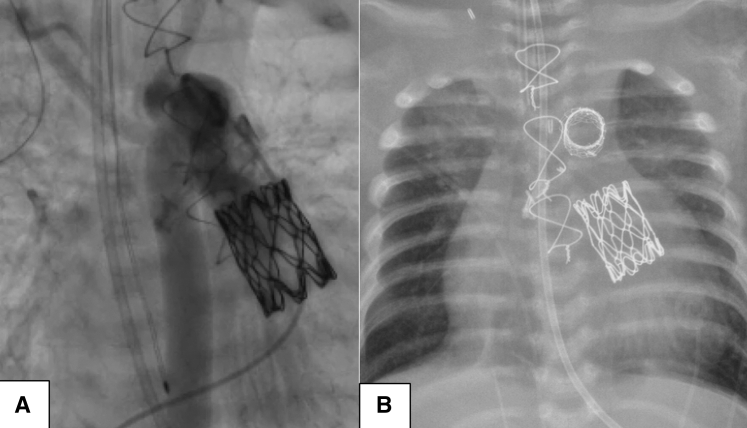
A, Postoperative cardiac catheterization showing well-seated 22-mm Melody valve in the pulmonary position with no insufficiency. B, Chest x-ray showing patent ductus arteriosus stent and 22-mm Melody valve.
